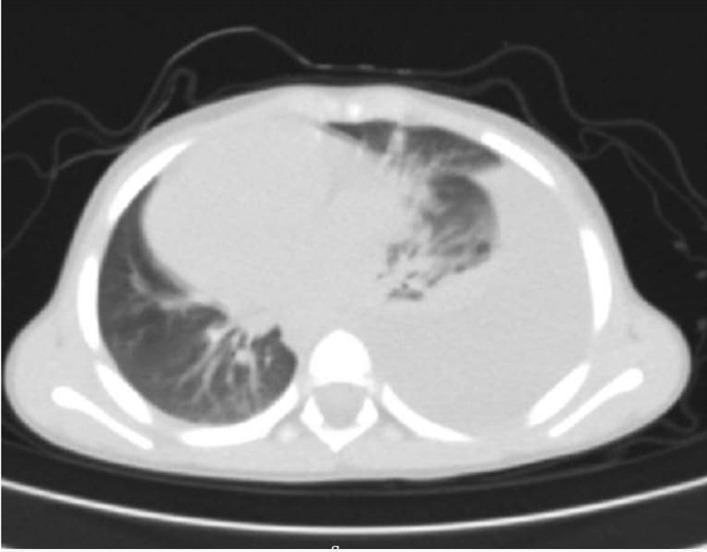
Computed tomography showing right-sided pleural effusion
